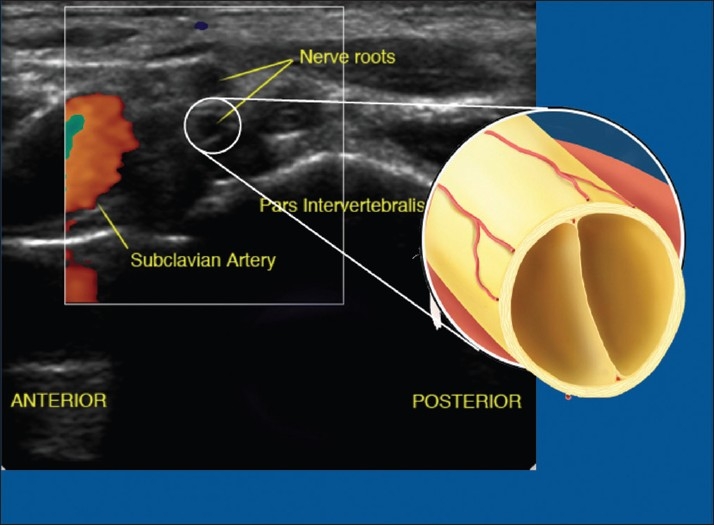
Ultrasound picture at the brachial plexus root level. Note root appears hypo-echoic
